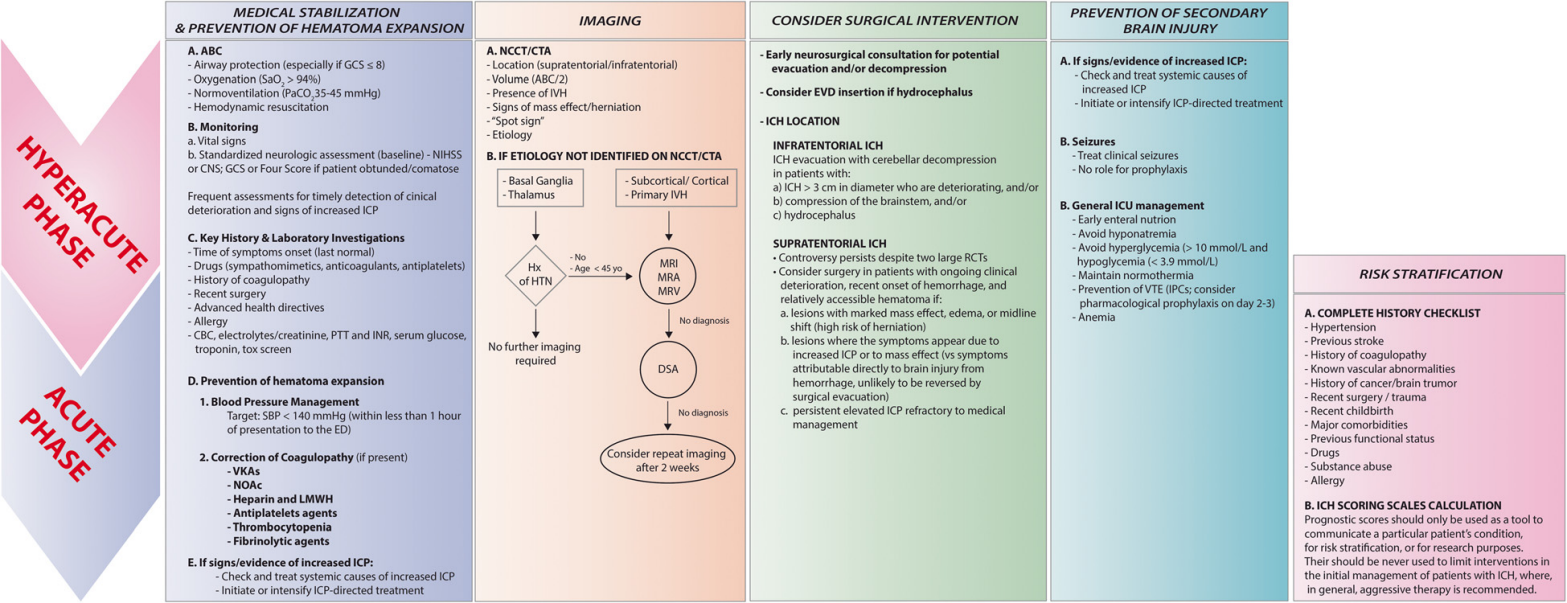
Principles of ICH management. *GCS* Glasgow Coma Scale, *SaO2* Oxygen arterial saturation, *PaCO2* partial pressure of carbon dyoxide, *ICP* intracranial pressure, *CBC* Complete Blood Count, *PTT* Partial Thromboplastin Time, *INR* international normalised ratio, *VKAs* Vitamin K inhibitors, *NOACs* newer oral anticoagulants, *LMWH* lower molecular weight heparin, *HTN* hypertension, *NCCT* non contrast computed tomography, *CTA* computed tomography angiography, *MRI* magnetic resonance imaging, *MRA* Magnetic Resonance Angiography, *MRV* Magnetic Resonance Venogram, *DSA* digital subtraction angiography, *ICH* intracerebral hemorrhage, *IVH* intraventricular hemorrhage, *NIHSS* National Institutes of Health Stroke Scale, *SBP* systolic blood pressure, *EVD* external ventricular drain
